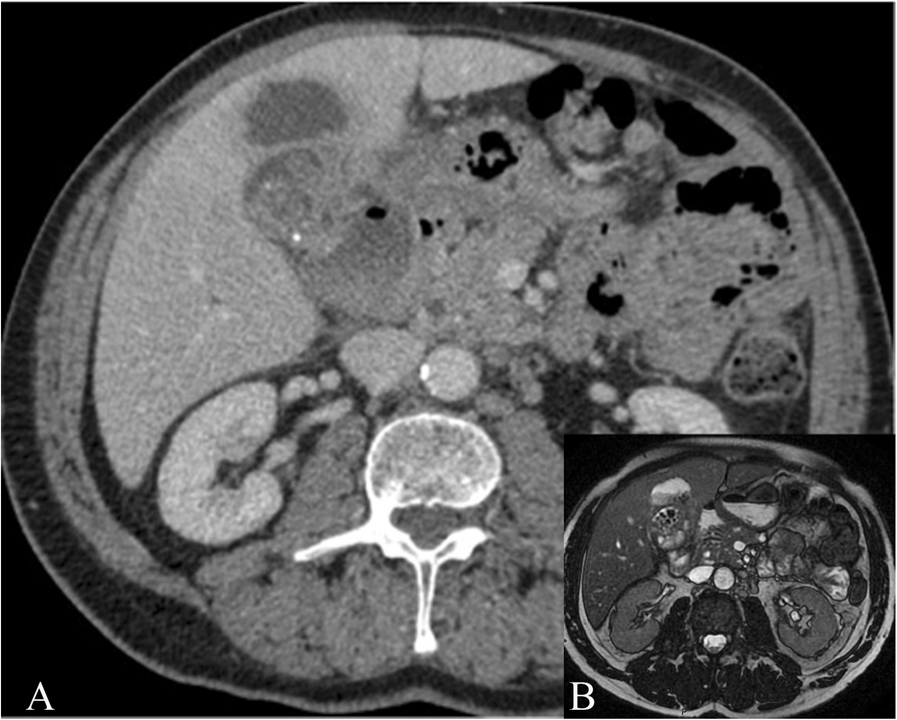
Preoperative Diagnostic. (A) Preop.-CT scan. A hypodense pseudocystic mass on the 5th liver segment, mimicking abscessed gallbladder cancer (bile stones are recognizable on the hilar part of the tumor). (B) MRI scan. Bubbles of free air inside the “tumour”.
